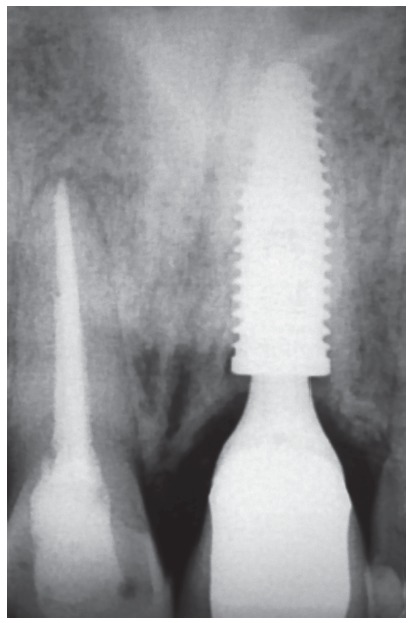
Radiographic aspects of the implant in the region of the decoronated tooth, six years after the procedure. Remnants of the tooth with replacement resorption and osseointegration are also noted.
